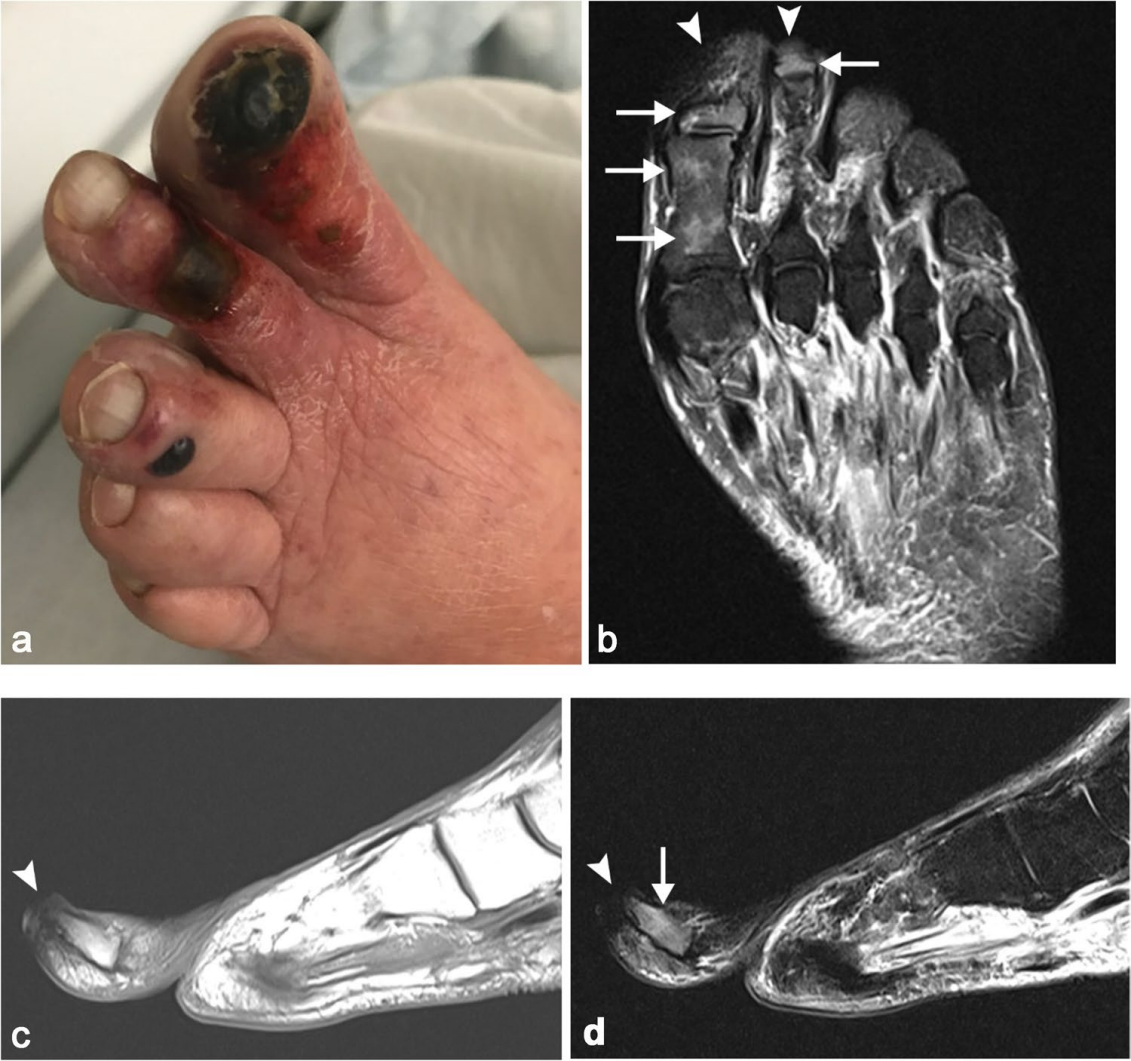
Eighty-five-year-old man with peripheral arterial disease with worsening pain and discoloration of his left toes. Clinical photograph (a) demonstrates areas of gangrene of his first, second, and third toes. Long-axis STIR (b), sagittal T1 (c), and STIR (d) MR images demonstrate signal loss of the soft tissues in the areas of gangrene (*arrowheads*) with patchy STIR hyperintensity (*arrows*) but normal T1 signal intensity within portions of the first proximal and distal and second distal phalanges, compatible with vascular insufficiency without superimposed infection (dry gangrene)
